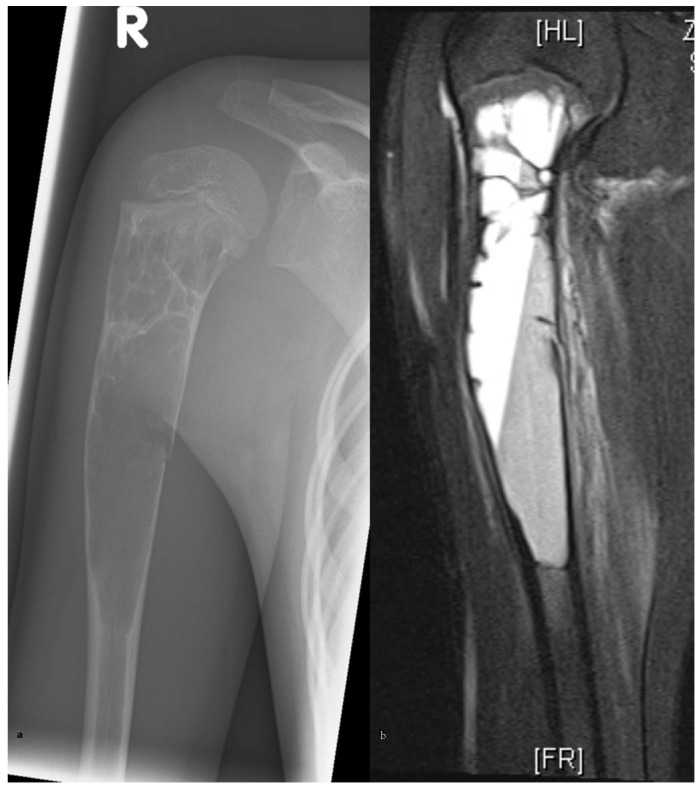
X-ray of the proximal humerus showing a bone cyst with a pathological fracture (a). Contrast-enhanced MRI displaying the characteristic fluid–fluid level (b).
